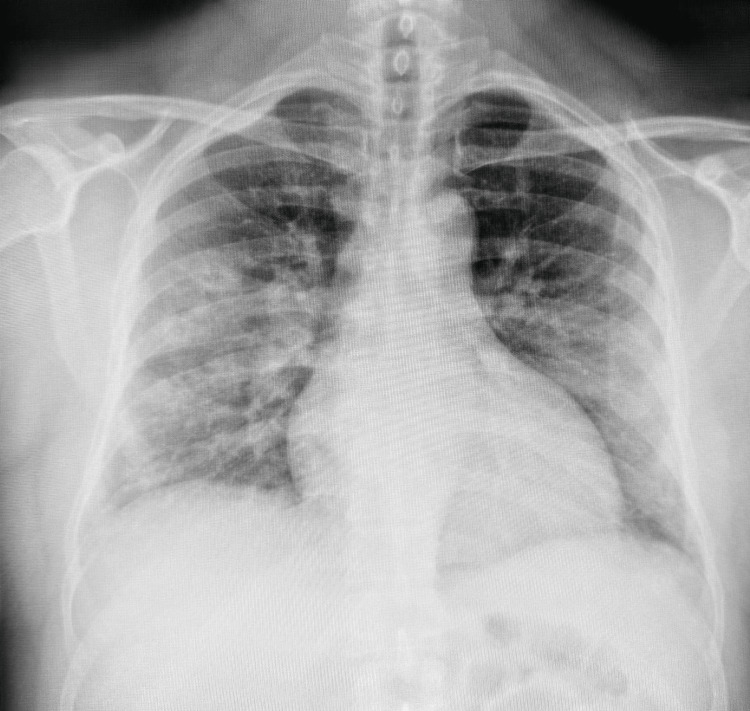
Chest radiograph Bilateral non-specific lung opacities with cardiomegaly.
